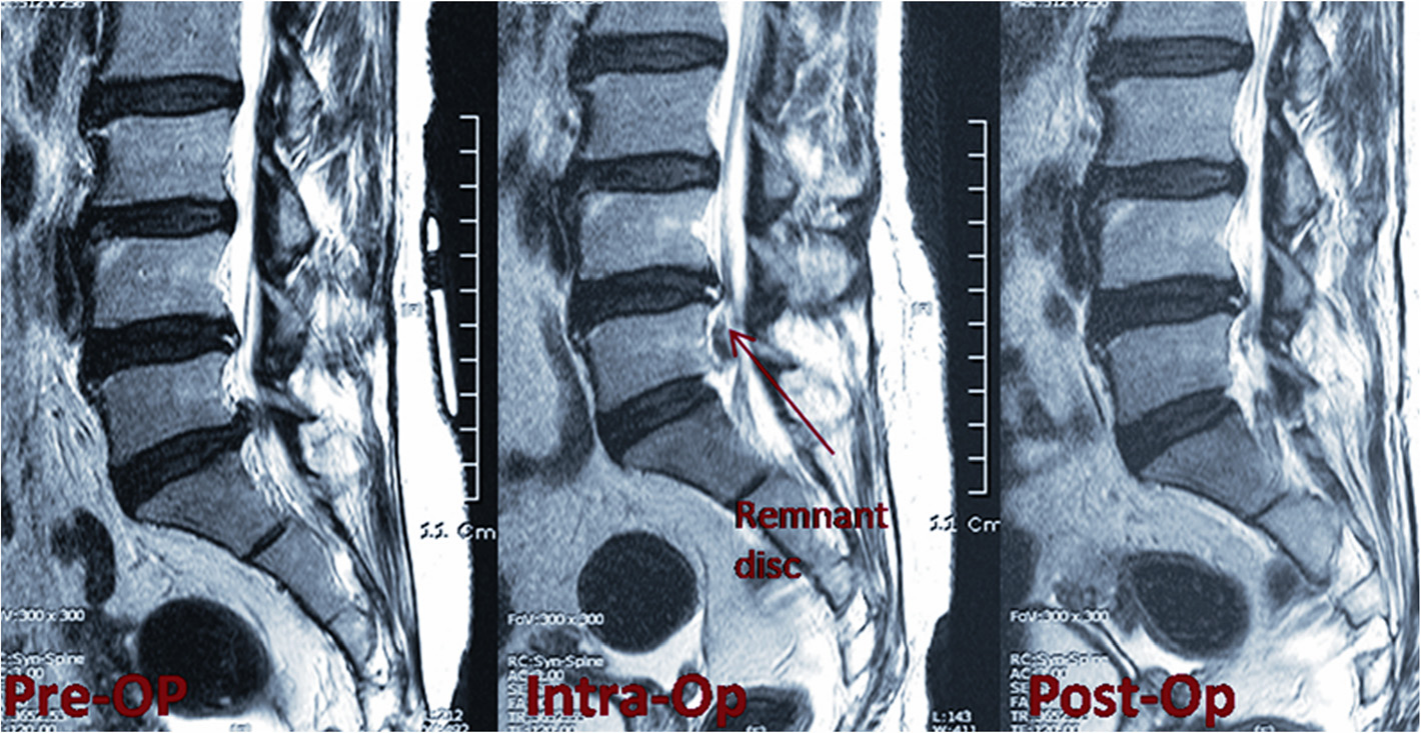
Intraoperative XMR confirming the remnant fragments and final MRI showing complete decompression.
